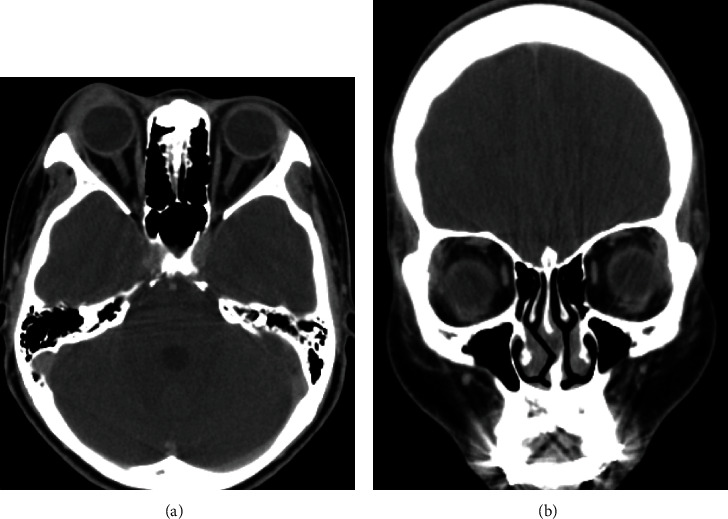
(a) Computed tomography (CT) axial view. (b) Computed tomography (CT) coronal view. (a, b) An infiltrative mass at the right superolateral orbital involving the lacrimal gland and upper eyelid.
